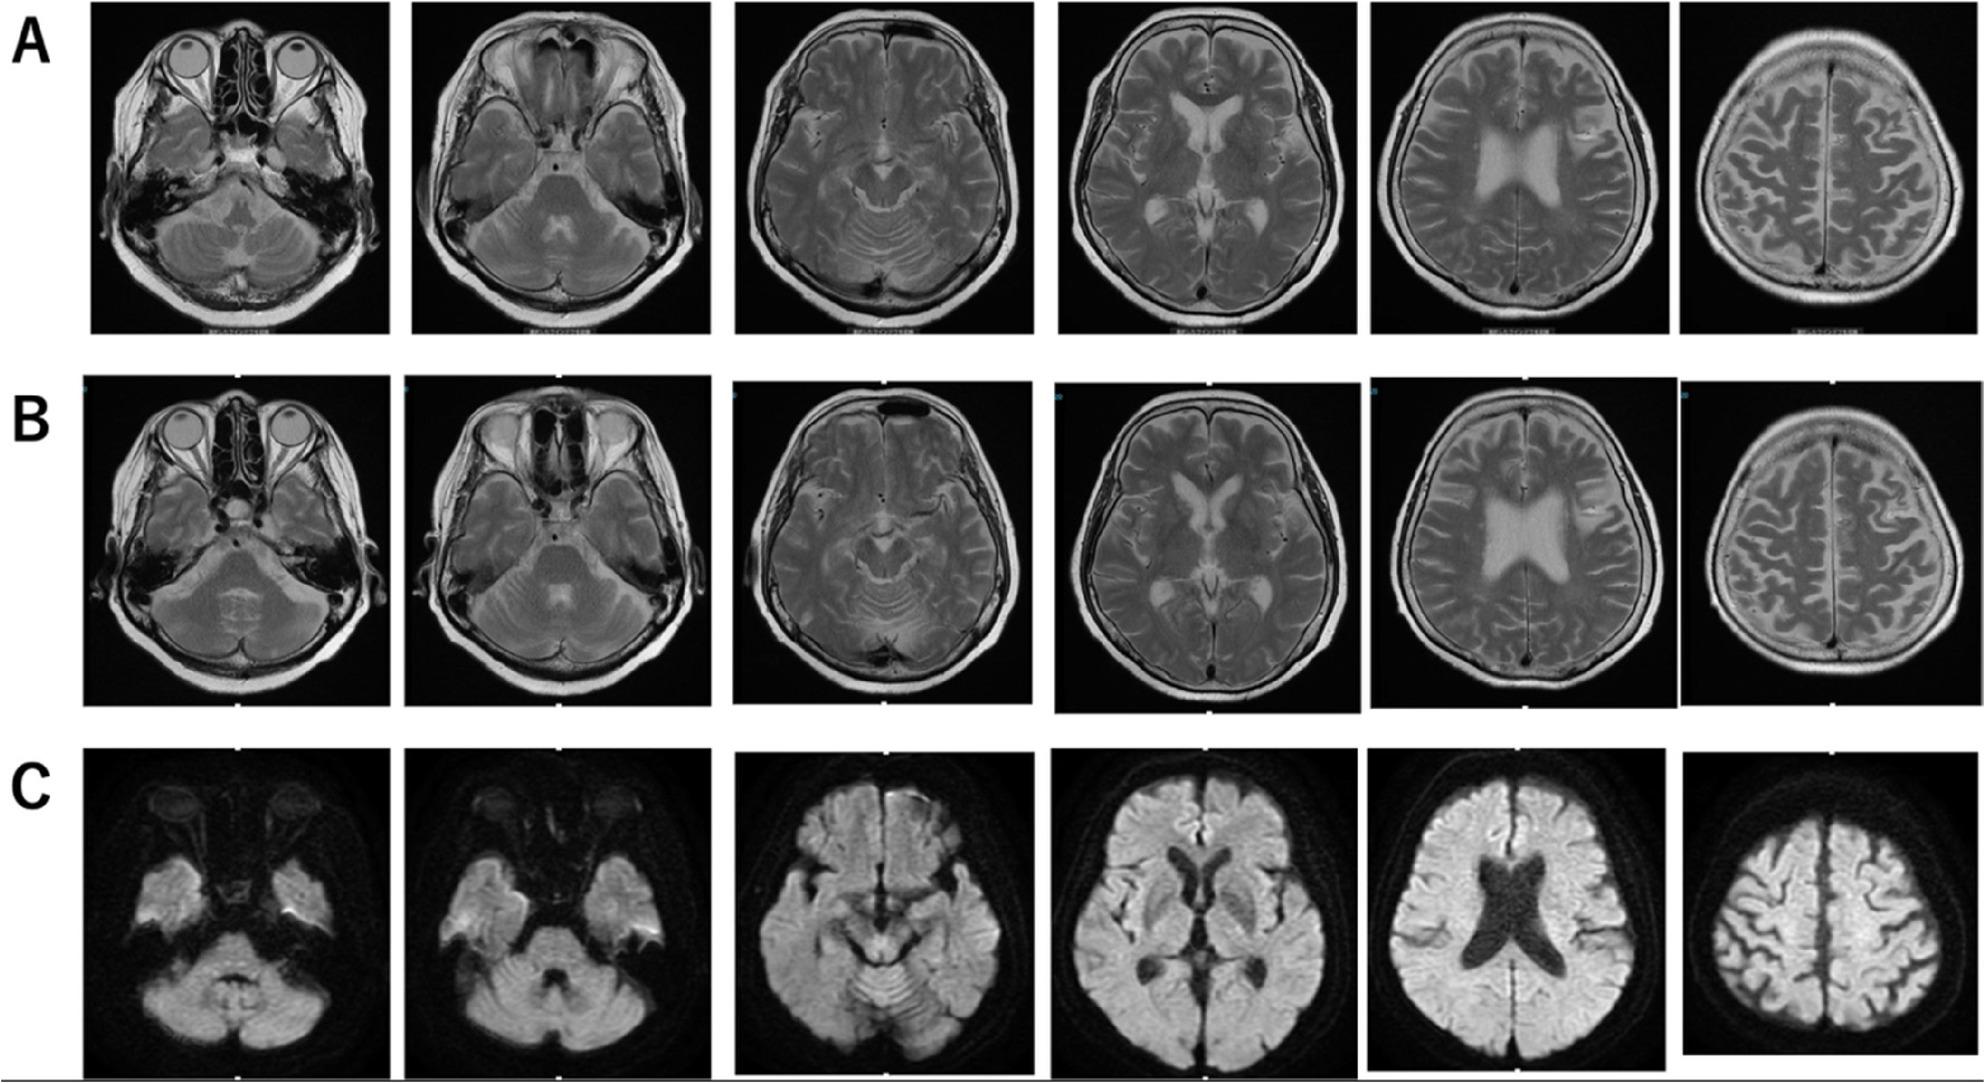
A Preoperative cerebral magnetic resonance imaging (MRI), T2-weighted sequence. B Postoperative MRI, T2-weighted sequence. C Postoperative MRI, diffusion-weighted imaging. Postoperative imaging demonstrated no new cerebral infarction, except for a pre-existing chronic subcortical infarct in the left frontal lobe
